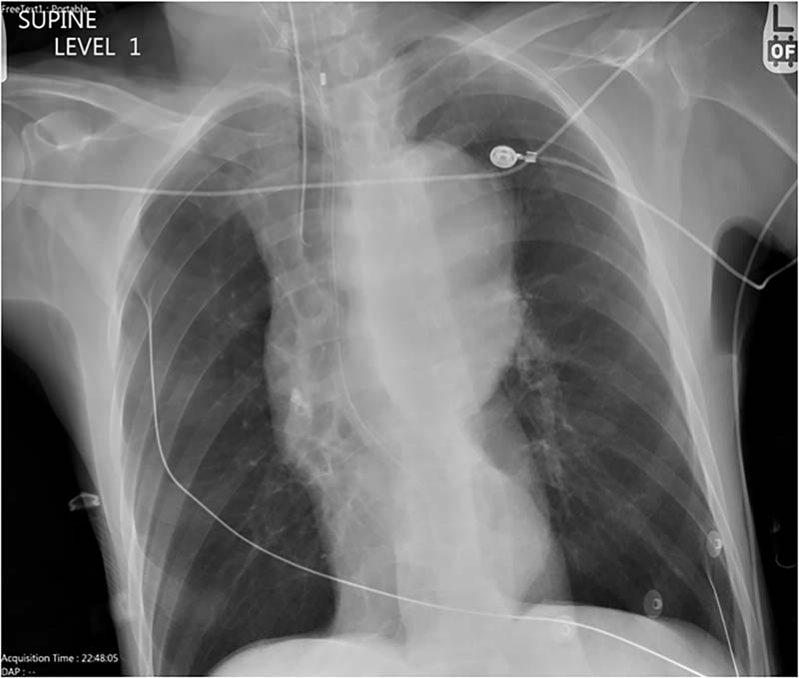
Chest X-ray with patient supine showing an impressive dilated thoracic aorta consistent with a large thoracic aneurysm.
